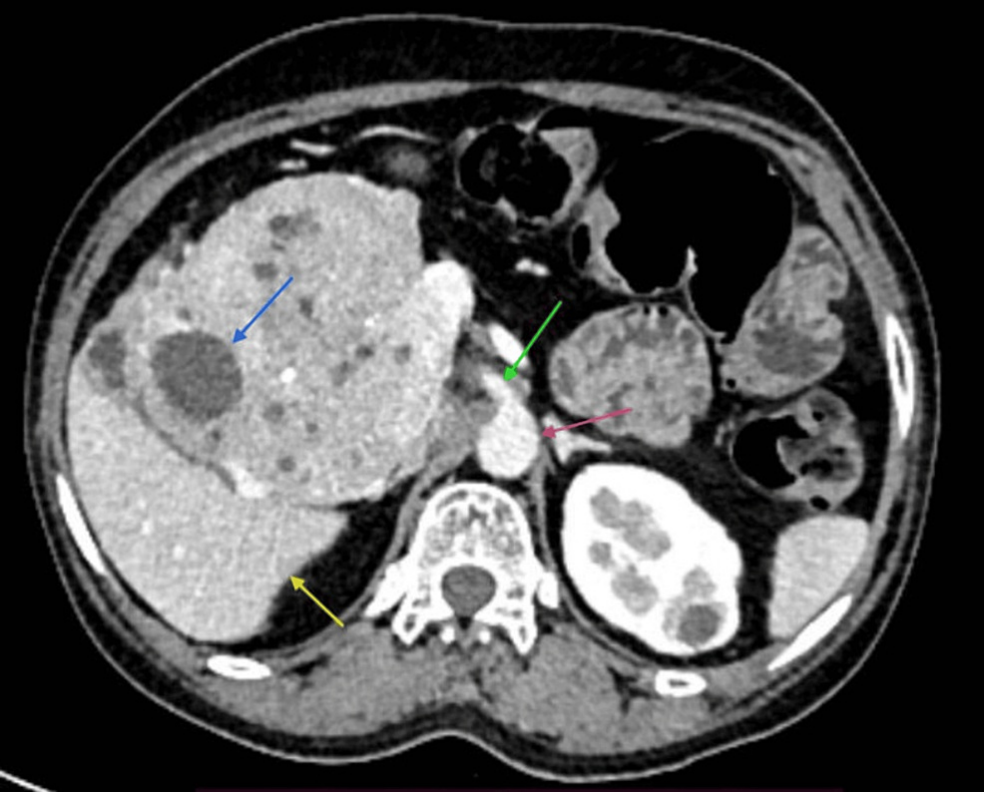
Triple-phase abdomen CT showing cystic pancreatic lesion. The red arrow points out the aorta, the green arrow points to the superior mesenteric artery, the blue arrow points to a cystic lesion of the pancreas, and the yellow arrow points to the liver.
